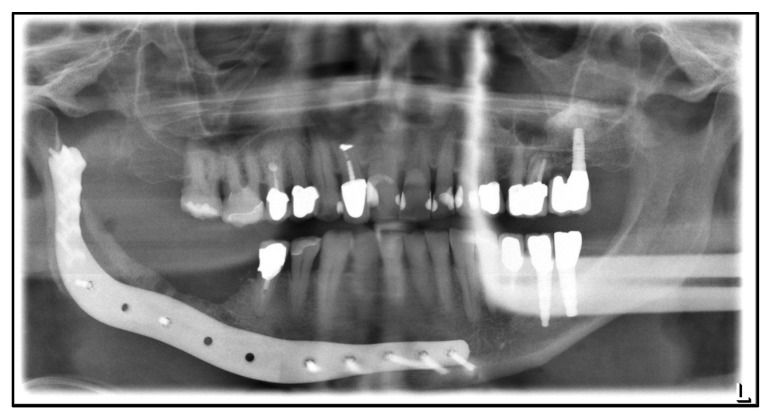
Dental X-ray after mandibular box resection and protective osteosynthesis, July 2014.
